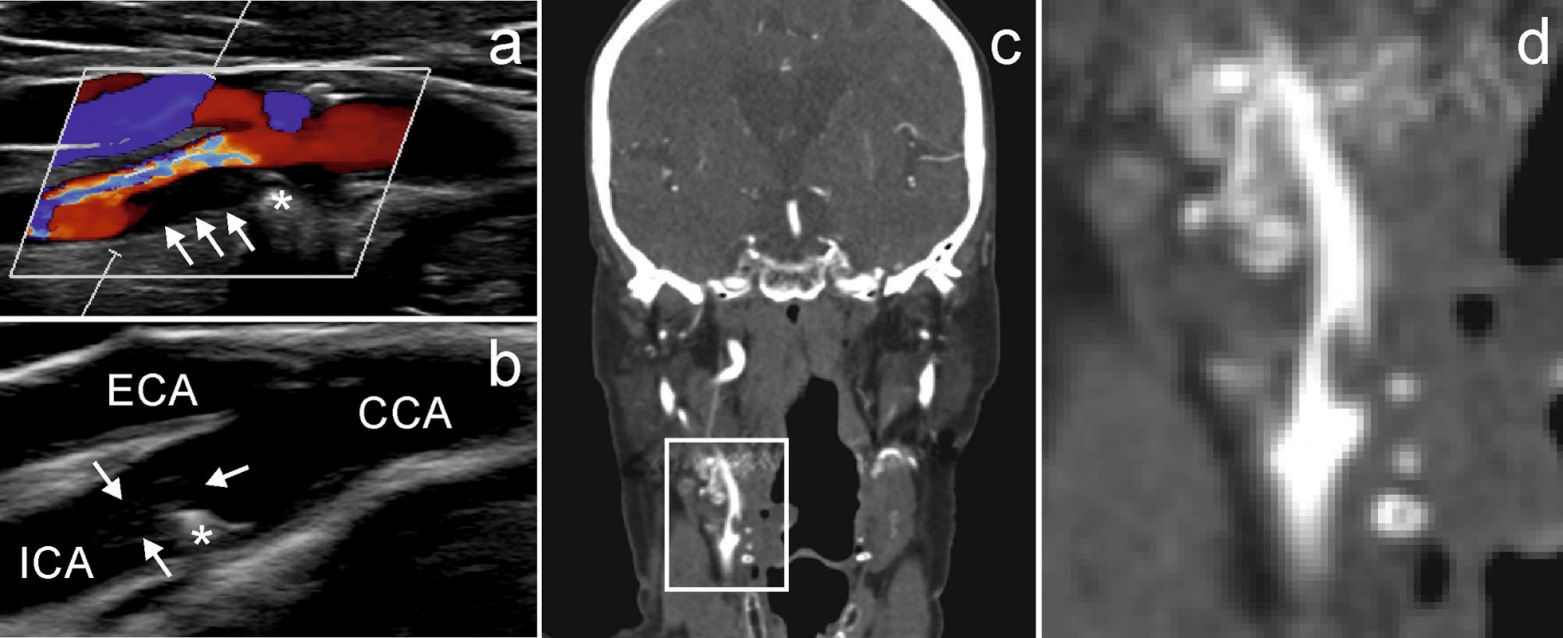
Example of an agglutinative thrombus a: Duplex sonography outlined stenosis (*) in the right ICA of a 90-year-old woman suffering from multiple embolic strokes located in the ipsilateral cerebral hemisphere. A hypoechogenic structure was attached to the stenosis (arrows). b: In the course of several control examinations, the structure shifted into the lumen of the ICA. c: This finding was confirmed by CT-angiography. d: Detail enlargement of picture c. The patient underwent a thromboendarterectomy and an agglutinative thrombus was extracted. CCA: common carotid artery; ECA: external carotid artery; ICA: internal carotid artery
